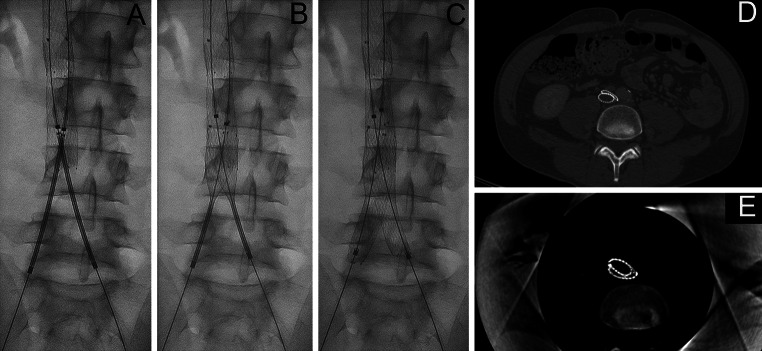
Confluence stenting with self-expandable stents (SECS). A–C self-expandable stents are placed within the large diameter IVC stent, extending into the common and/or external iliac veins. D CT shows compression of one iliac self-expandable stent, while the adjacent stent shows adequate expansion. E per-operative conebeam CT shows another example of significant compression by the contralateral stent
